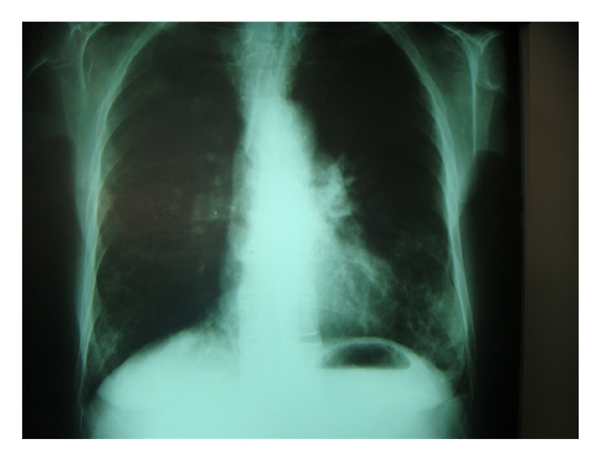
Chest X-ray: infiltration in the base of both lungs.
